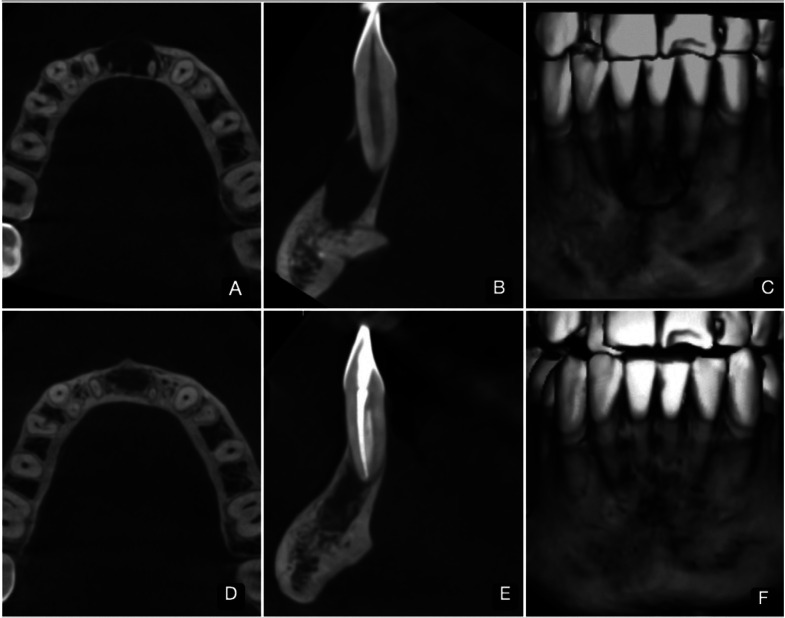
CBCT images of Clinical Case #1. A-B-C; Axial and sagittal view and three-dimensional reconstruction of the pre-treatment state. D-E-F; Axial and sagittal view and three-dimensional reconstruction of the post-treatment state after 6 months. Considerable diminution of the mesio-distal width can be observed, with peripheral areas of bone neoformation, recovery of lingual plate thickness and complete closure of the vestibular fenestration.
